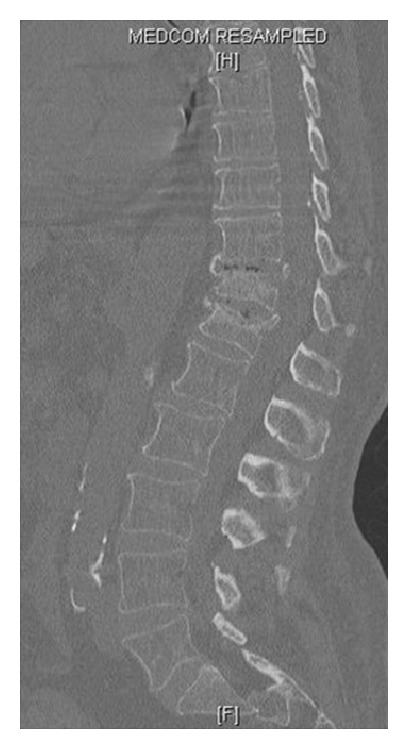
Preoperative sagittal CT scan of a 72-year-old male with T11 and T12 fractures.
